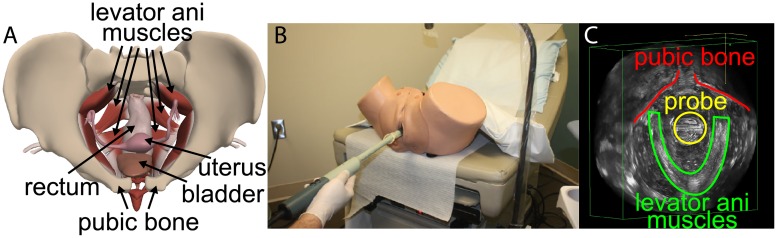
The anatomy of the women pelvic floor. (A) Axial view. Pelvic floor muscles are the layer of muscles that support pelvic floor organs, assist in urinary and fecal continence and stabilize connecting joints. (B) 3D Endovaginal Ultrasound Imaging with 360° rotational transducer using a BK Ultrasound System (Flex Focus 500) [9]. (C) Pelvic floor muscle appears as a V-shaped structure in 3DEVUS (green boundary). Pubic bone and probe can also be viewed interactively during acquisition and offline.
